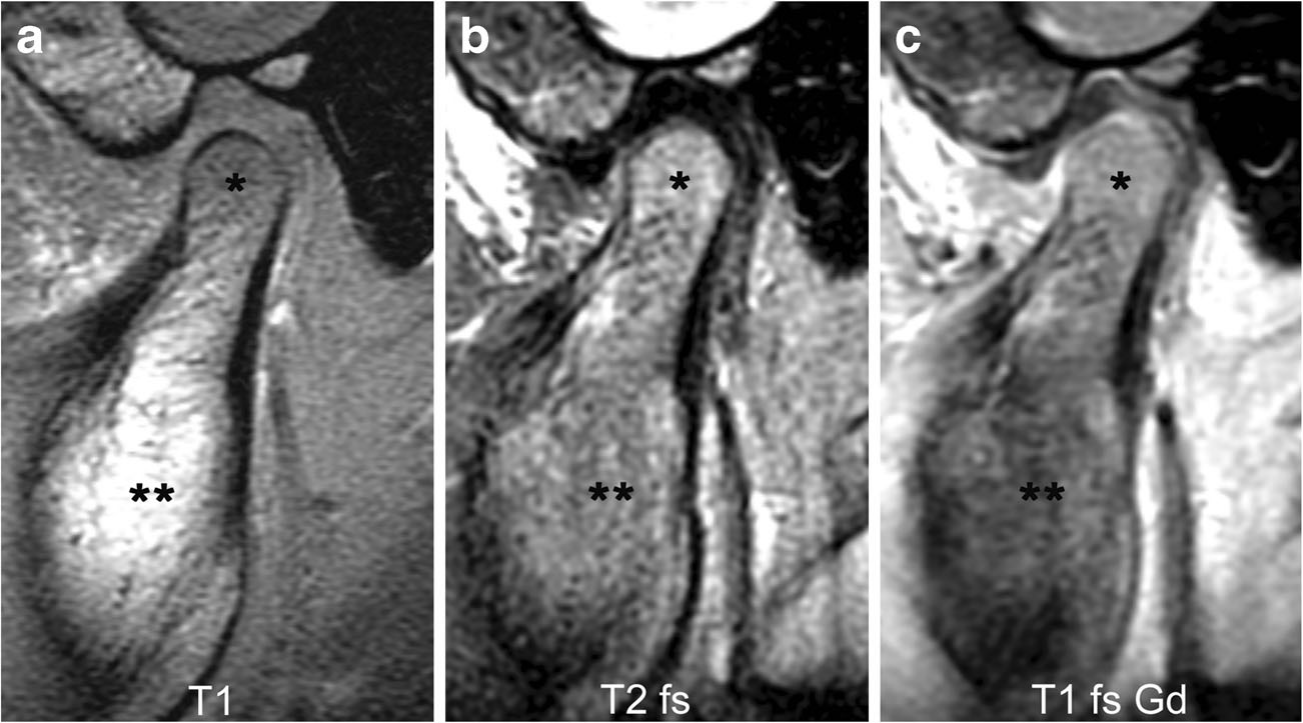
Bone marrow oedema and enhancement (grade 1) of the mandibular condyle in a 9-year-old boy. a-c When compared to the mandibular ramus () there is lower signal from the mandibular condyle (*) on the T1-weighted gradient echo image without fat saturation (TR/TE 300/4.2 ms, flip angle 80°, a), and slightly increased signal on fat-saturated T2-weighted image (TR/TE 5,400/77 ms, b) and on postcontrast fat-saturated T1-weighted image (TR/TE 670/10 ms, c) images, Note mild joint enhancement with high signal intensity (hyperintense to muscle) in the posterior superior joint recess and lower joint compartment on postcontrast fat-saturated T1-weighted image (c)
